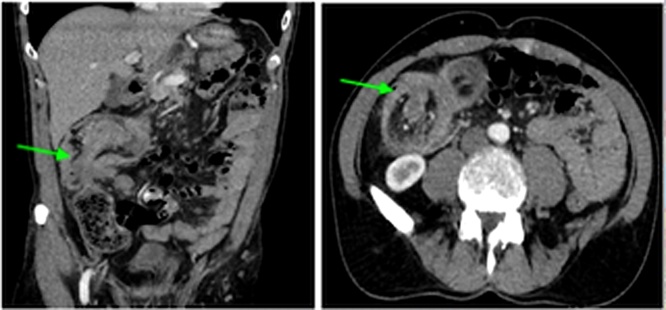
Initial contrast-enhanced computed tomography scans of the abdomen demonstrating colon-colonic intussusception of the cecum and ascending colon.
